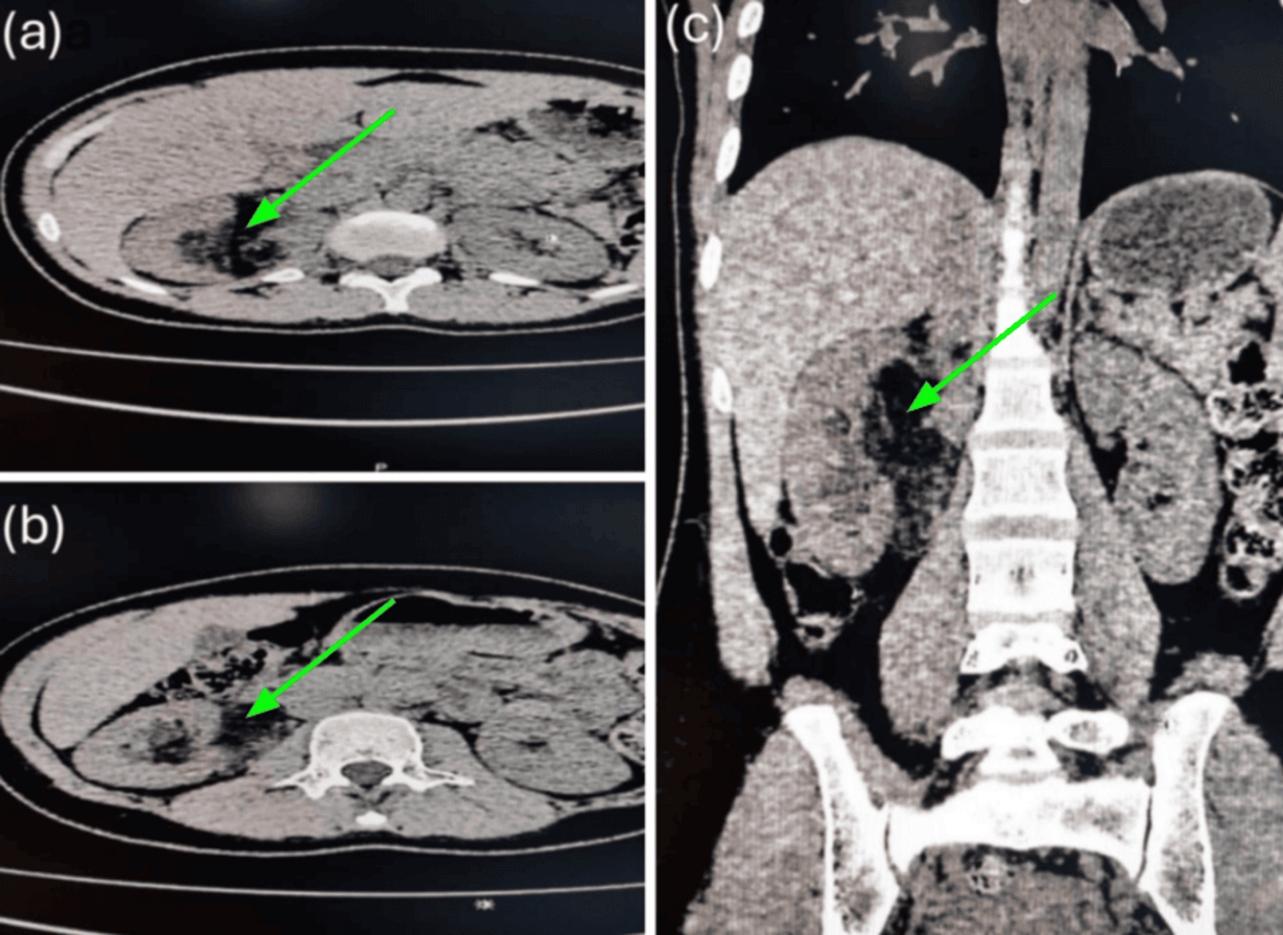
(A) CT KUB axial image showing a large, irregular, ill-defined, heterogeneous, predominantly fat-attenuating lesion with focal areas of soft tissue attenuation involving the pelvicalyceal system of the right kidney. (B) CT KUB axial image demonstrating extension of the lesion into the dilated renal pelvis. (C) CT KUB coronal reformatted image showing an abrupt transition at the right pelvicalyceal junction, suggestive of ureteropelvic junction obstruction with mild to moderate dilatation of the right pelvicalyceal system. KUB, kidneys, ureters, and bladder
